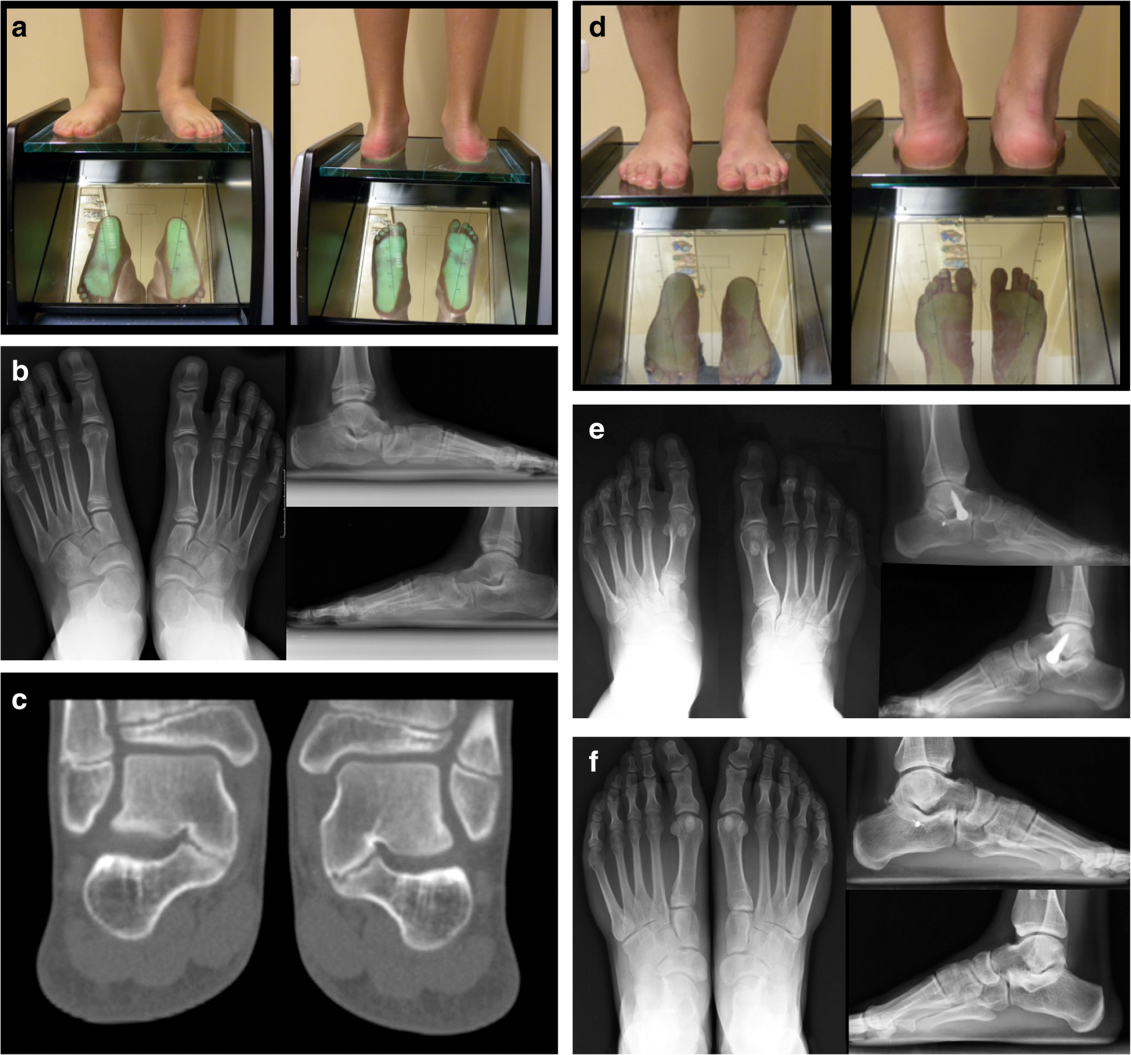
Clinical and radiographic features of 12 years old boy with RFF and TCC. a Clinical aspect on podoscope. b Antero-posterior and lateral radiographs of the same patient showing the collapse of the longitudinal arch, hindfoot valgus, and forefoot abduction. The “talar beak”, evident on the neck of the talus, suggests the presence of TCC. c Coronal CT scan of both feet showing “type I” TCC according to the Rozansky’s classification. d Post-operative clinical aspect on podoscope 1 month after surgery. e Radiographic aspect showing the screw arthroeresis with correction of the flatfoot. f Radiographs 6 years after screw removal, showing that the correction is maintained, and the radiographic parameters are restored
